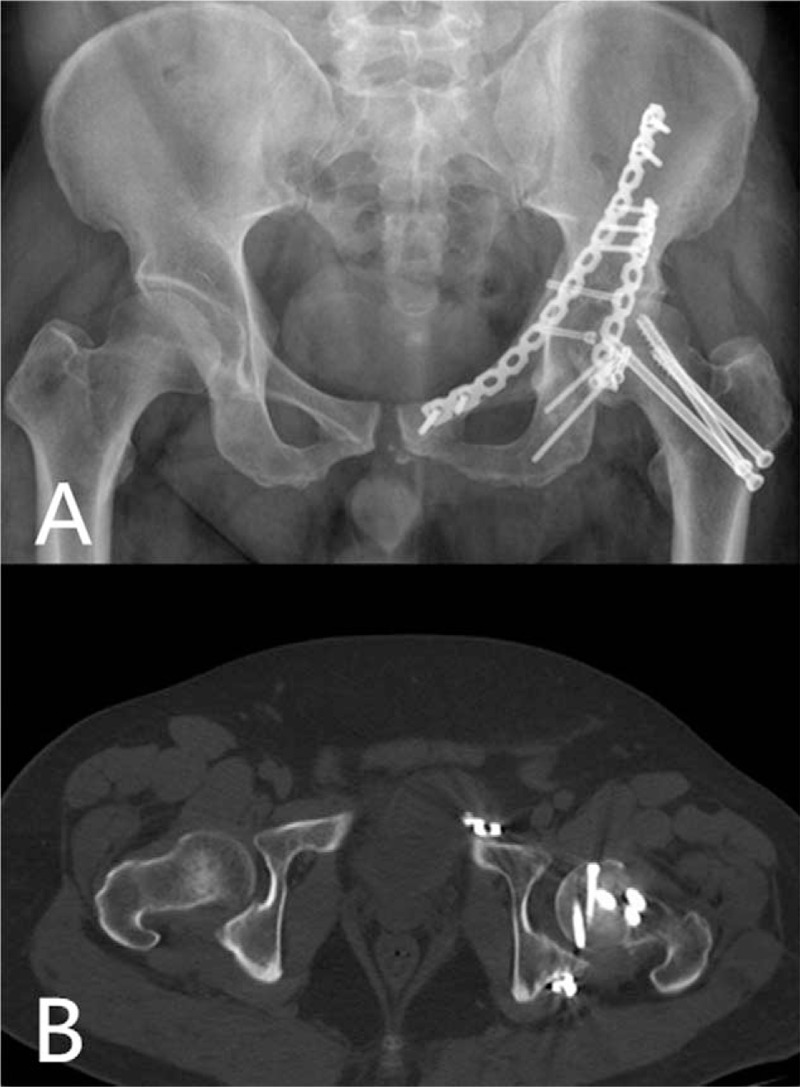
(A and B) Anteroposterior x-ray and CT images showing union of fractures with no femoral necrosis signs. CT = computed tomography.
